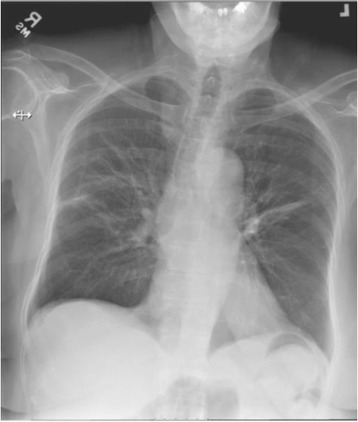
Patient’s chest X-ray after stopping methotrexate and starting steroids
